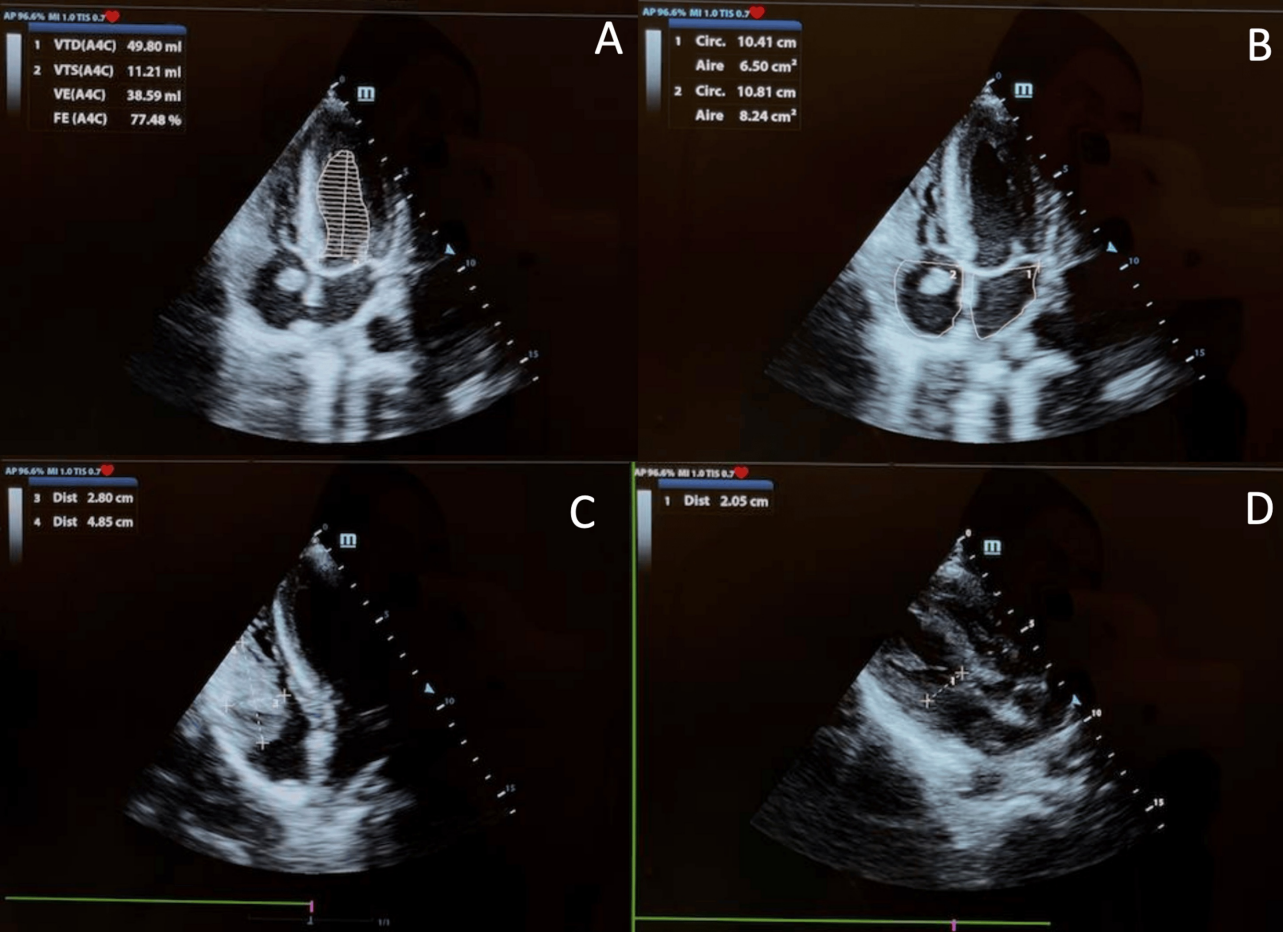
Echocardiography: RA and RV masses confirming MRI features; tumor thrombus suspected. (A) Preserved systolic function (LVEF 77%). (B) Normal atrial areas and a visible right atrial mass. (C) Extension into the right ventricle. (D) Preserved right ventricular size. Images were obtained from the authors’ institutional database. RA, right atrium; RV, right ventricle; MRI, magnetic resonance imaging; LVEF, left ventricular ejection fraction
